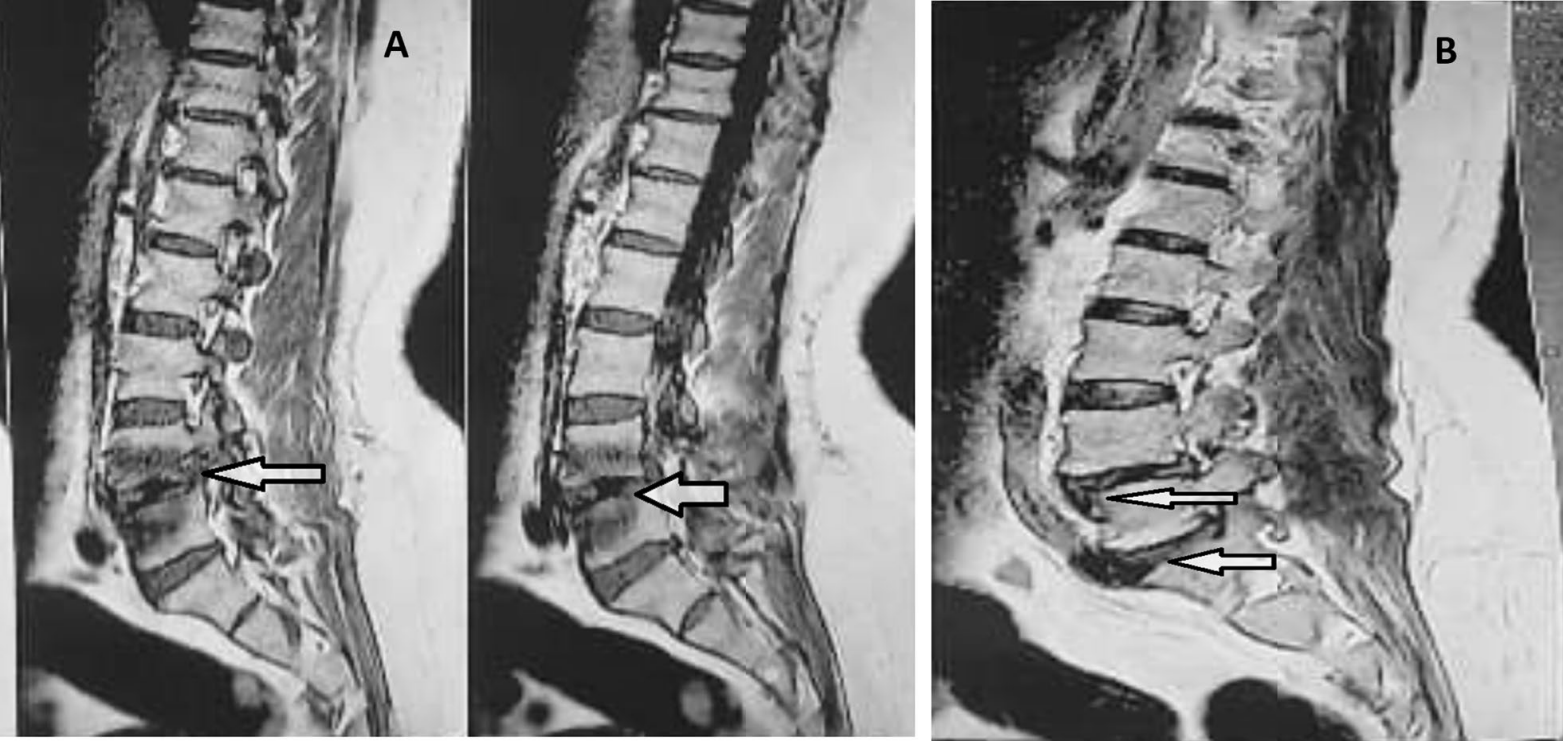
A MRI depicting the discitis involvement around L4-L5, B discitis involvement around L4-L5 (Up arrow) and discitis involvement around L5/S1 (Down arrow)
